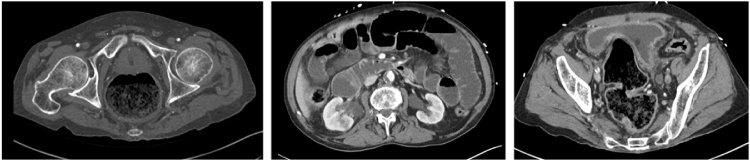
Computed tomography at admission. Left panel: Shows fecaloma and thickened rectal wall; Middle panel: Shows dilated bowel loops with no transition point; and Right panel: Shows distended bladder and edematous wall.
